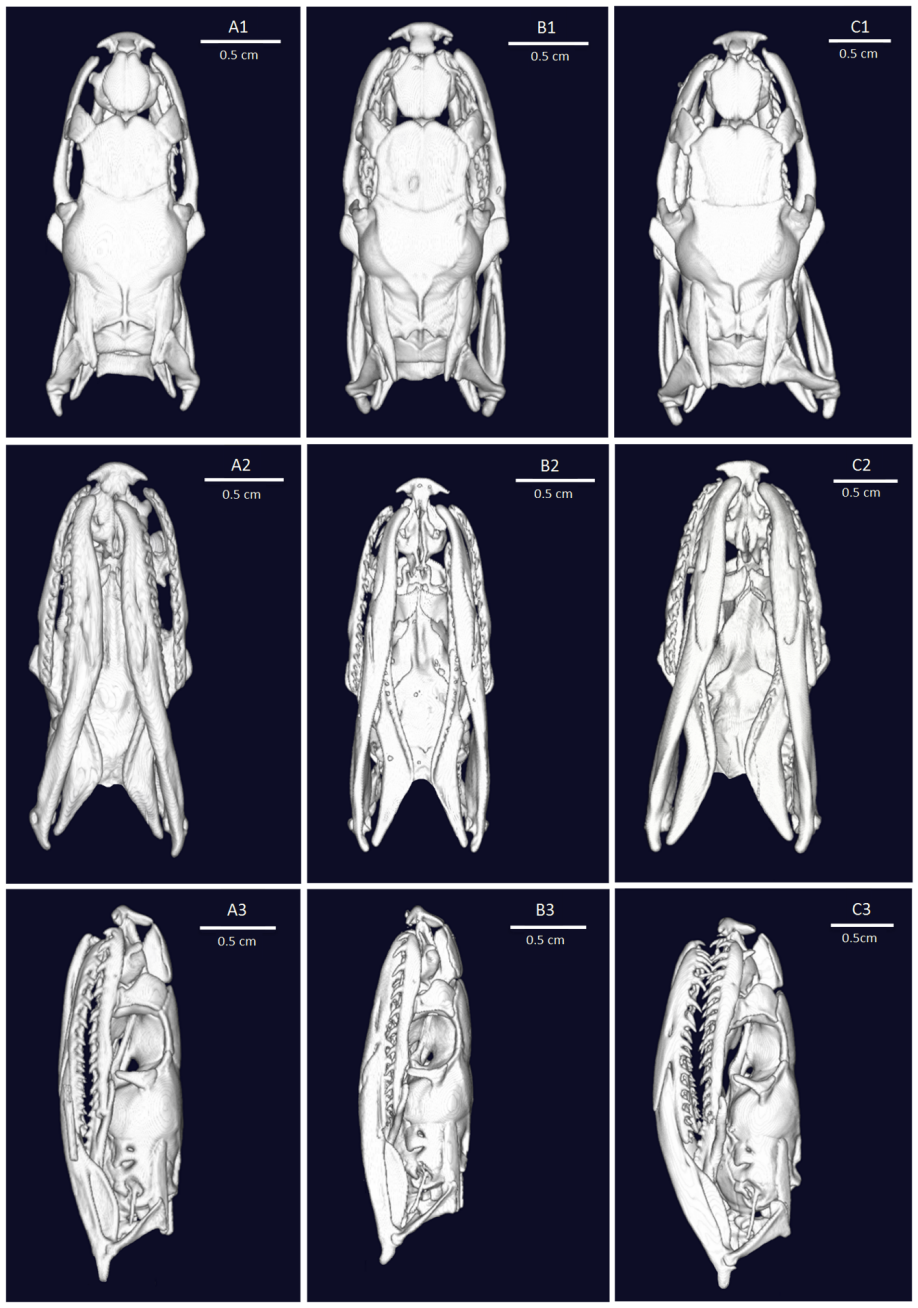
CT scans of skulls of *Oreocryptophis porphyraceus* from Yunnan (A: *O. p. pulchra*), Zhejiang (B: *O. p. vaillanti*), and Hainan (C: *O. p. hainana*)
